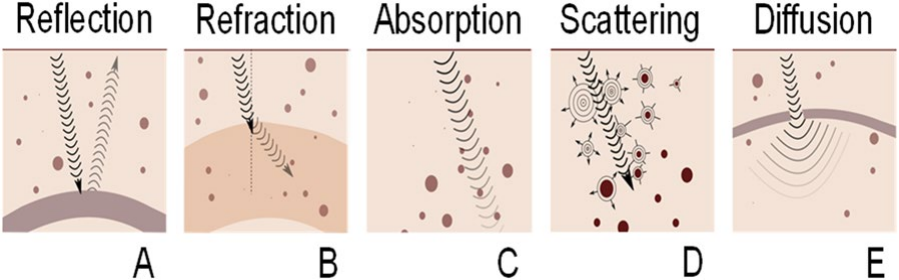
During their propagation, compression or shear waves are modified due to their interaction with the physical medium. Their direction and amplitude may change due to (a) reflection or (b) refraction at the interface of media with different acoustic impedance. The amplitude may also decrease (i.e., attenuation) due to (c) absorption and (d) scattering produced by the insonified medium. e Space between scatterers or within specular reflectors favor the spreading of the wave field due to diffraction. Waves received by the ultrasound transducer to produce an image are attributed to reflection and scattering
